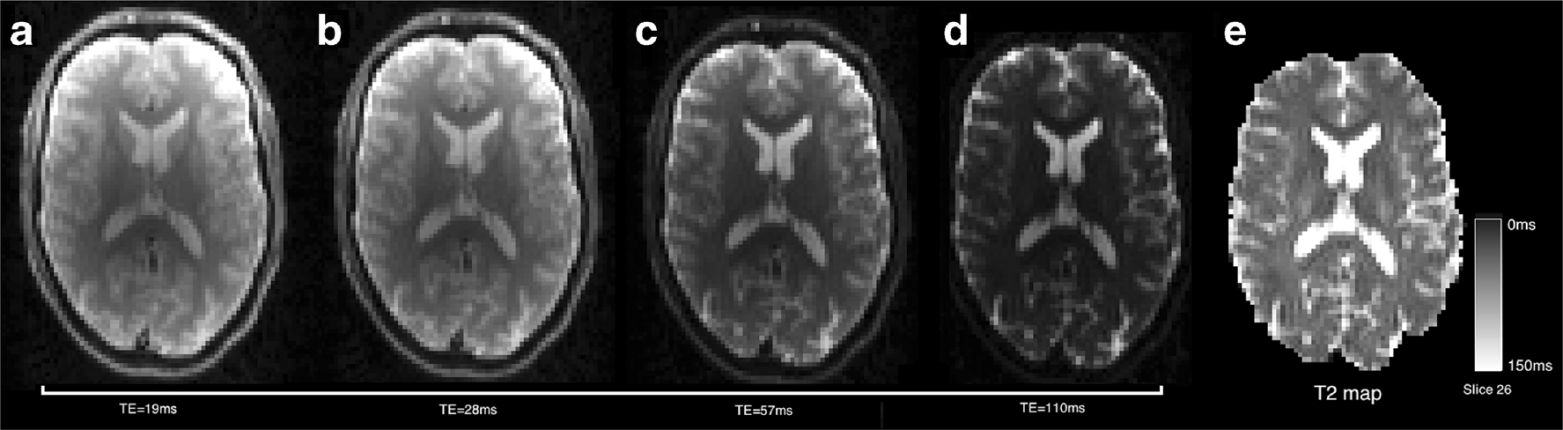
Example multi-echo T2-weighted acquisitions with four different echo times (a–d) and an estimated T2 map (e)
